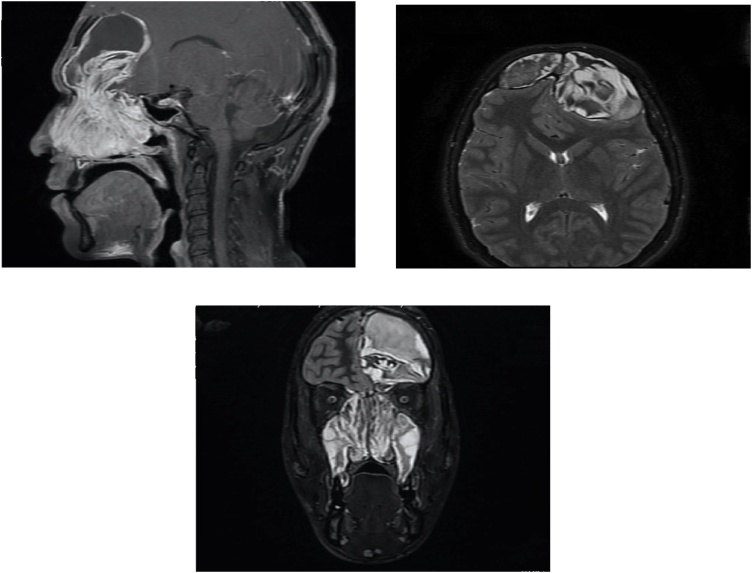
MRI of the brain and sinuses showed significant expansion of both frontal sinuses by the mucopyoceles.
